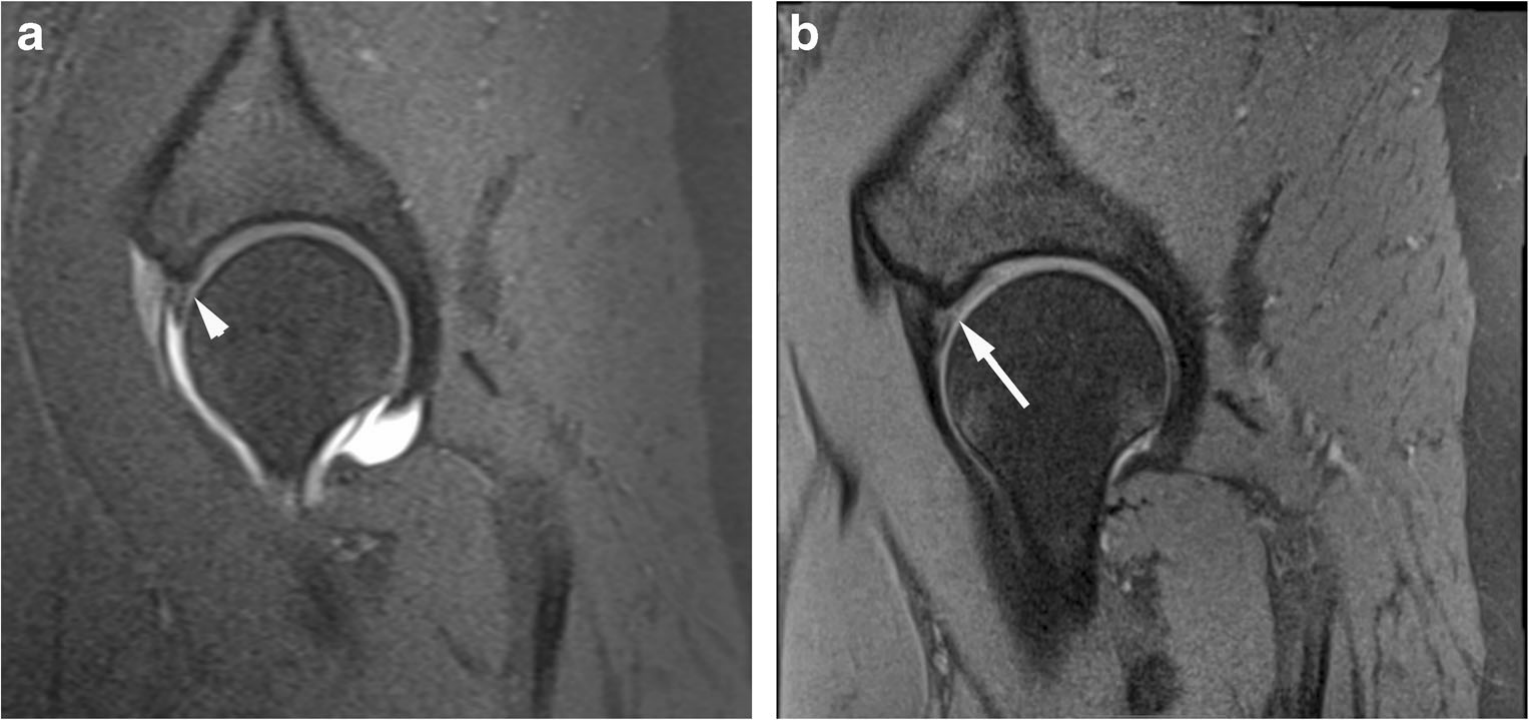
Anterosuperior partial thickness cartilage defect in a 32-year-old confirmed at surgery. Sagittal (a) T1 FS 1.5T MRA shows normal articular cartilage at the junction with the labrum (arrowhead) and (b) PD FS 3T MR image shows partial thickness cartilage defect (arrow)
